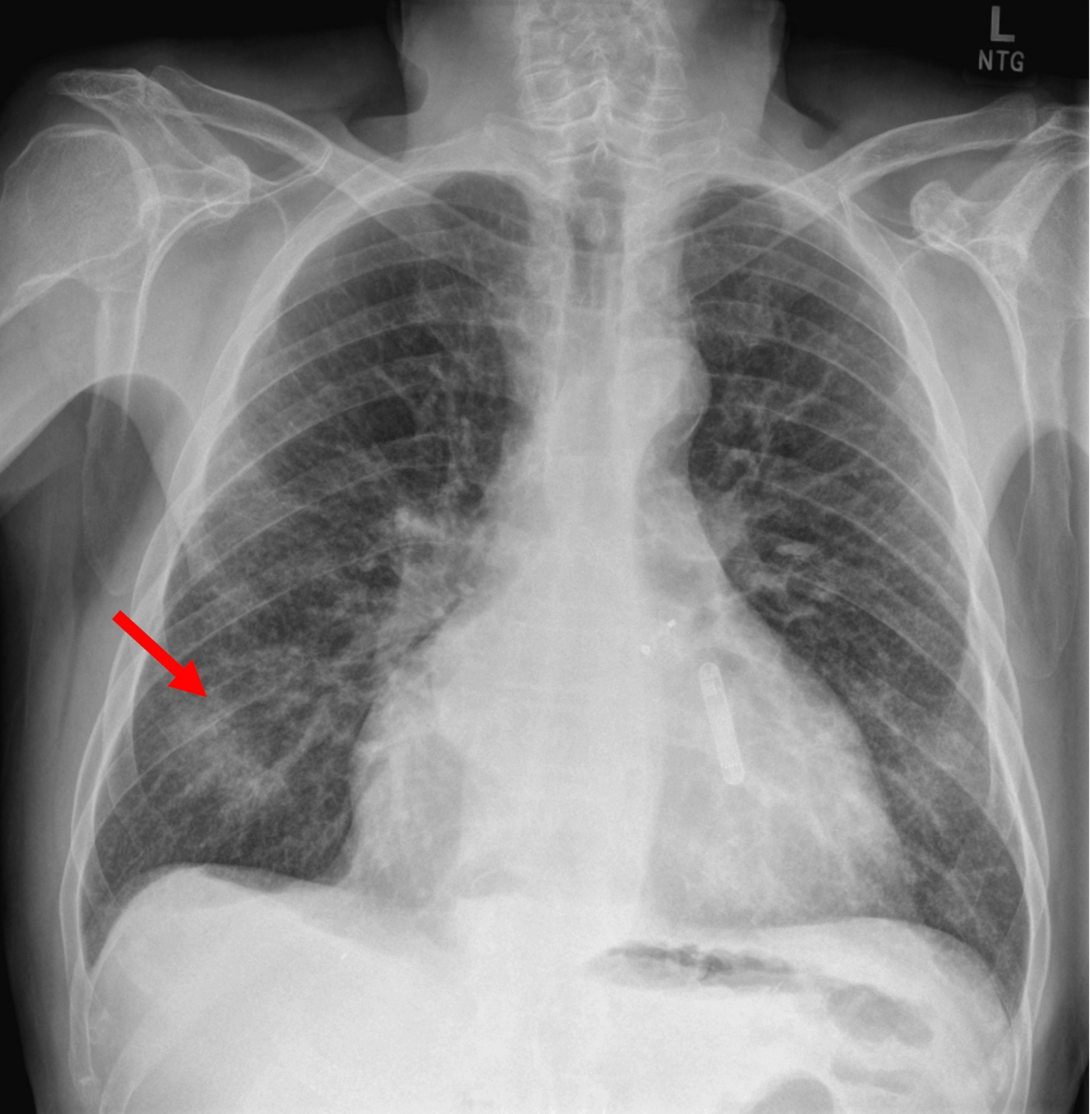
Chest X-ray with the red arrow denoting area of aspiration in the right lower lobe.
